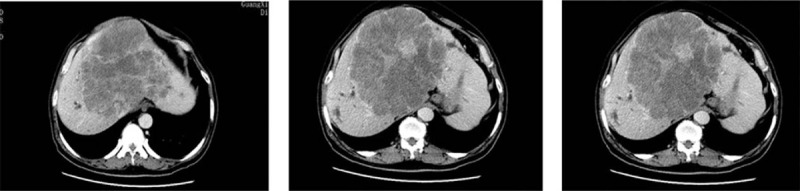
Computed tomography showed multiple hepatic metastases and a future liver remnant <30%.
